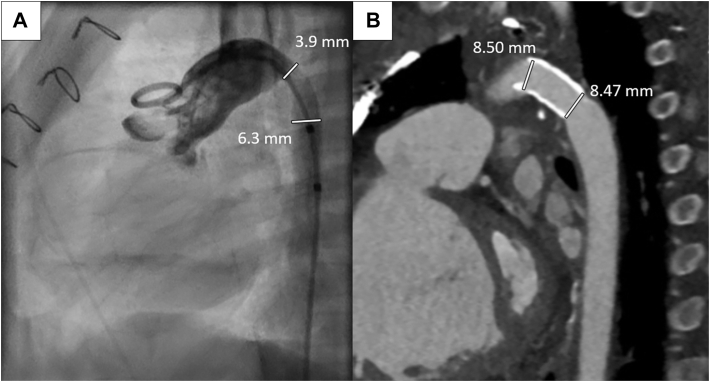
(A) Preoperative angiogram demonstrates the area of recurrent coarctation and (B) postoperative computed tomographic image with patent open hybrid stent.
